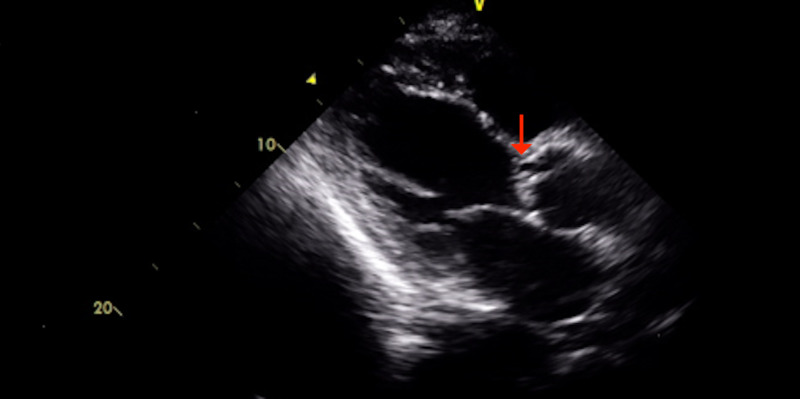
Parasternal long-axis view revealing the aortic valve vegetation and leaflet perforation.
